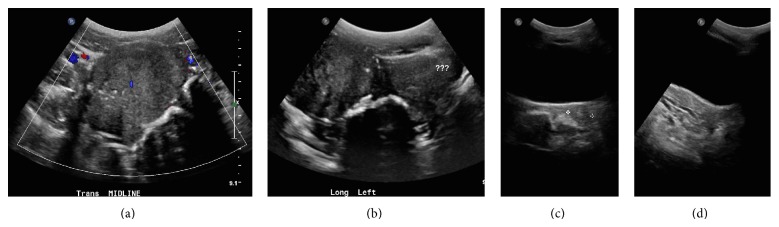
Transabdominal ultrasound imaging. (a), (b) Transverse and longitudinal images of large bilateral solid pelvic masses. Normal ovaries were not identified. (c), (d) Transverse and longitudinal images of a normal prepubertal uterus.
